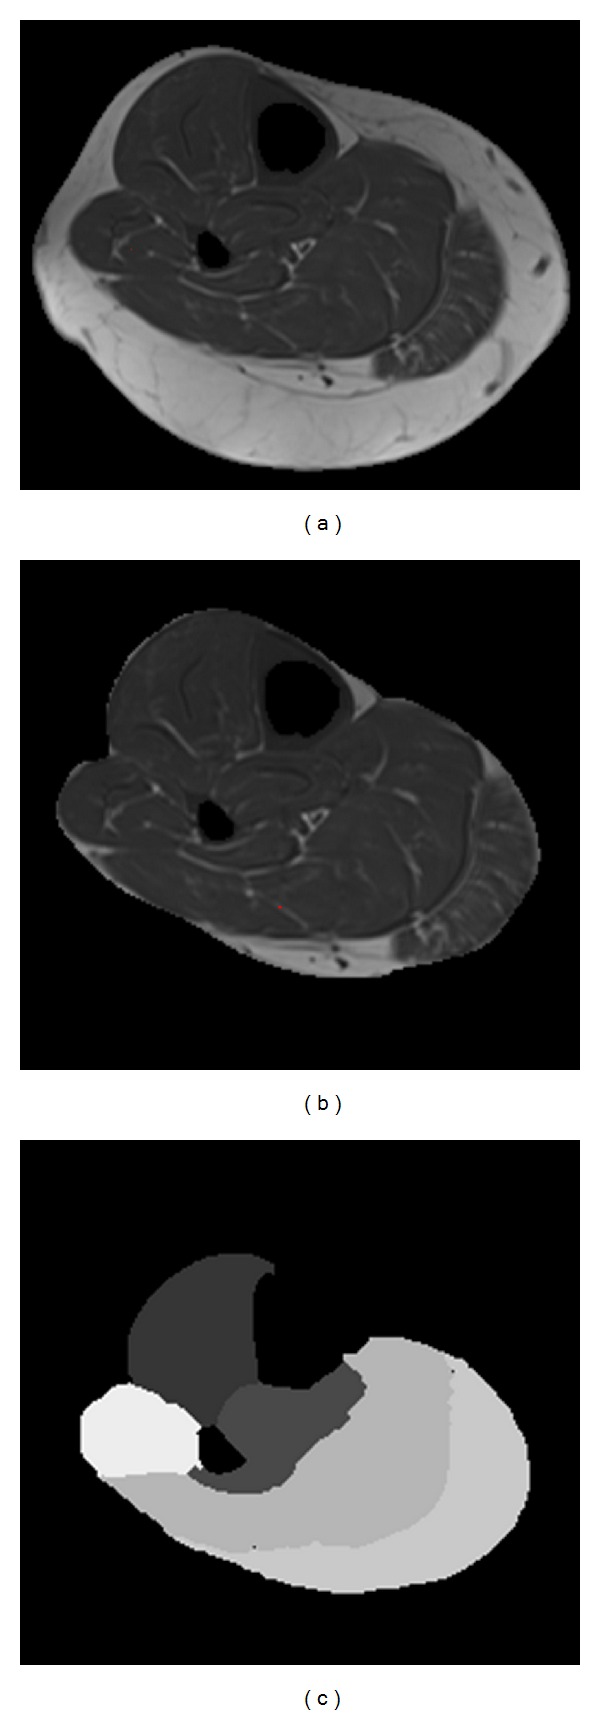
(a) MRI image of calf with bone removed. (b) Subcutaneous adipose tissue removed. (c) Calf divided into 5 compartments (anterior, lateral, deep, gastrocnemius, and soleus).
